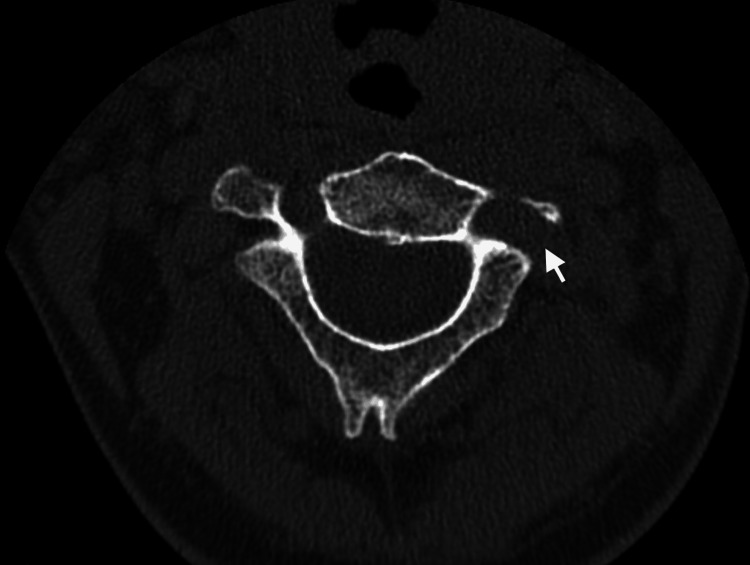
CT cervical spine showing the expanded C2 foramen transversarium
